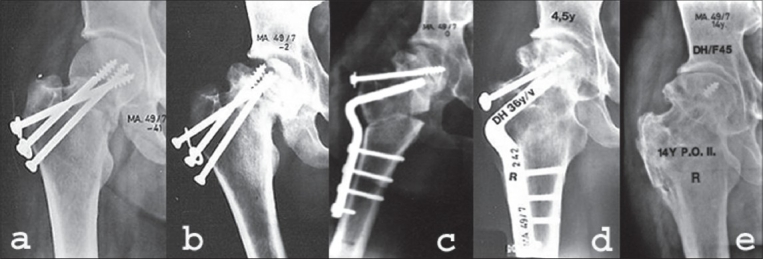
Female, 30 years. (a) Three months after screw fixation of a displaced femoral neck fracture shows healing fracture. The patient did not report for followup. (b) Two years after the primary fracture the patient was readmitted after a fall. She now seems to have a pathological fracture in the necrotic femoral head. (c) Postoperative X-ray after open reduction of the fracture was performed. The fracture was stabilized only by a screw and valgization osteotomy. (d) Healed osteotomy and fracture, 4.5 years postoperative. (e) Fourteen years after the osteotomy. There is little trochanteric pain, the patient walks one hour without any support
